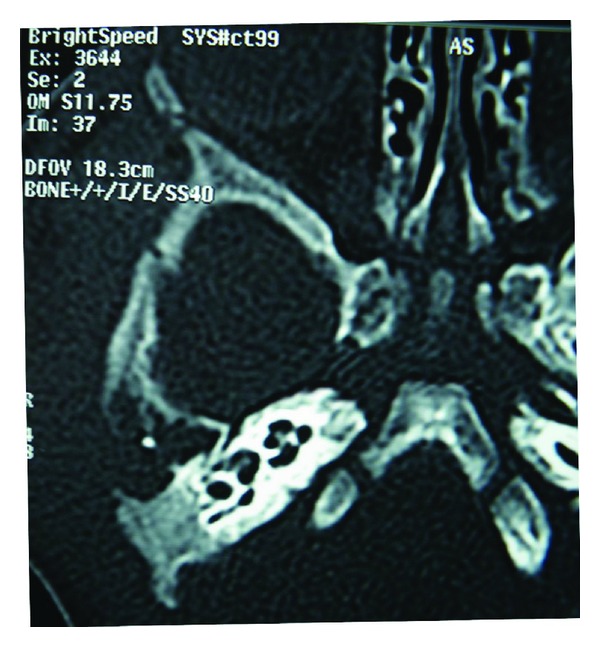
CT scan showing bony lytic lesions.
